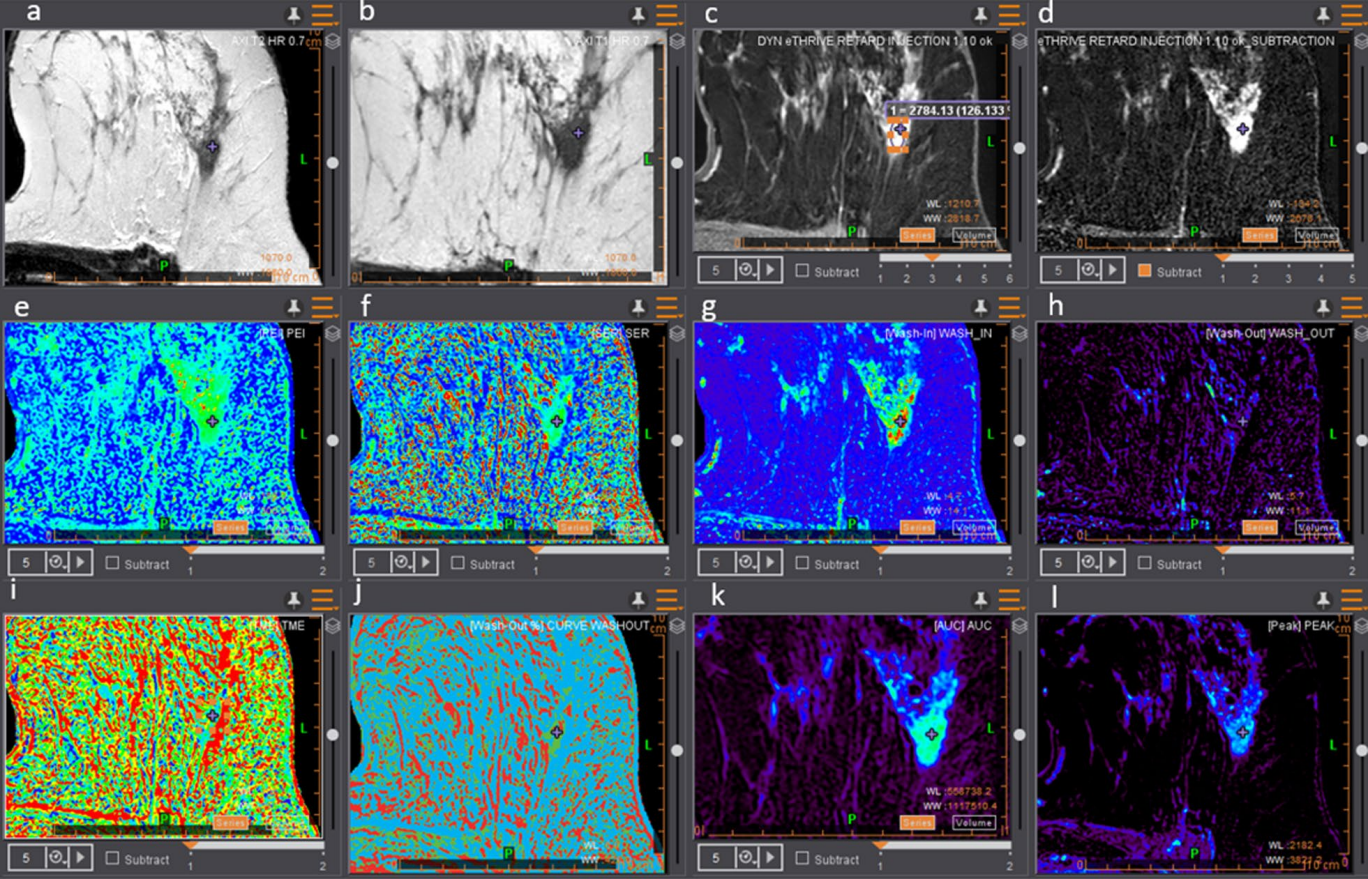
Example of a grade II infiltrating carcinoma of the left breast at the meeting point of the lateral quadrants: a T2-weighted sequence in axial view, b T1-weighted sequence in axial view, c Dynamic contrast enhancement 3 native, d Dynamic contrast enhancement 1 subtracted, e PEI map, f signal enhancement ratio map, g WASH IN map, h WASH OUT map, i TME map, j WASHOUT CURVE map, k AUC map, l Peak map
